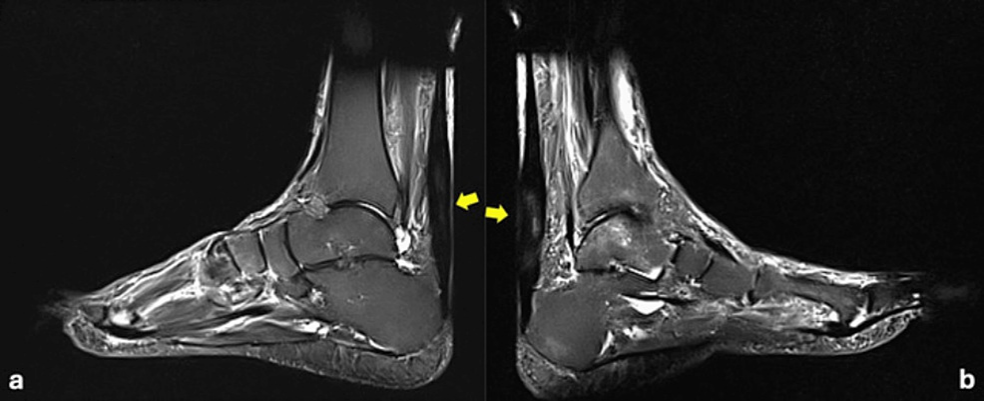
Three-month post-injury MRI of the right (a) and left (b) ankle showing successful healing of Achilles tendons (yellow arrows)
